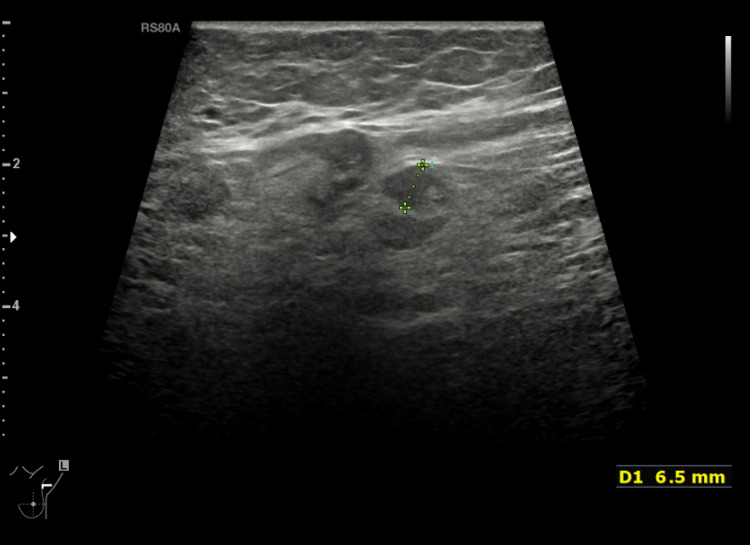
Left axillary ultrasound showed multiple abnormal-looking enlarged left axillary lymph nodes with loss of central fatty hilum and central vascularity noted.
